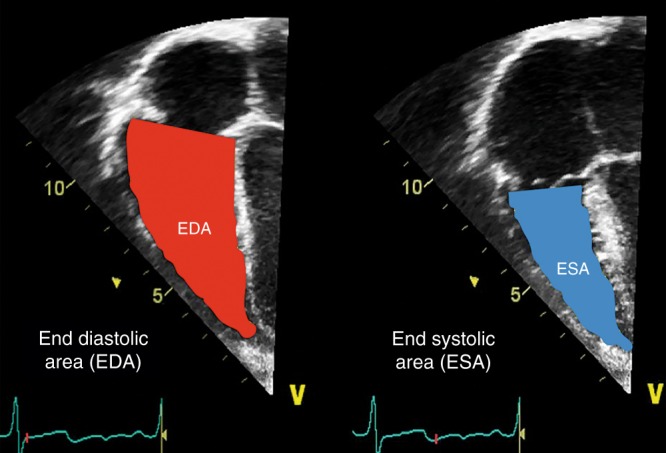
Fractional area change (FAC). FAC is a planimetric measure of the ratio of end systolic area (ESA) to end diastolic area (EDA) in apical 4- or 3-chamber view by manual tracing of the endocardial border of the right ventricle (FAC (%) = [EDA−ESA]/EDA). Trabeculation should be included within the cavity under the tracing procedure
